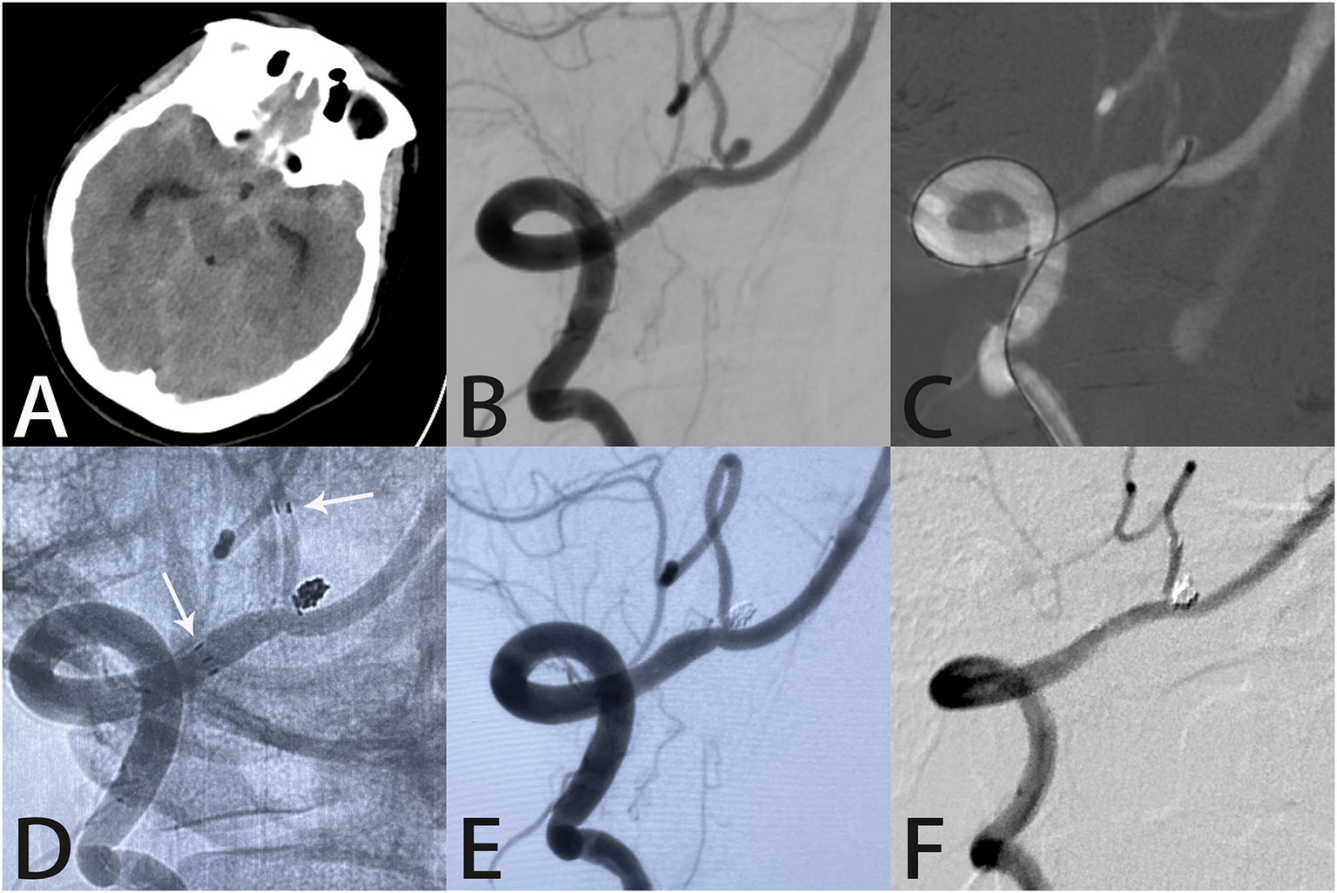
Images from a 45-year-old woman with a tiny ruptured right posterior inferior cerebellar artery aneurysm. Computed tomography showed subarachnoid hemorrhage in the lateral fissure and basal cisterns (A). Angiography demonstrated a right posterior inferior cerebellar artery aneurysm (B). A microcatheter was delivered into the aneurysm sac (C). An unsubtracted image showed the distal end of the Atlas stent (3.0 × 15 mm) was placed in the right posterior inferior cerebellar artery with the proximal end in the right vertebral artery (white arrows indicate the ends of the stent) (D). Angiography immediately after the procedure showed complete aneurysm occlusion and unobstructed blood flow in the posterior inferior cerebellar artery (E). Follow-up angiography 7 months later showed complete aneurysm occlusion with the coils densely packed within the aneurysm sac (F).
